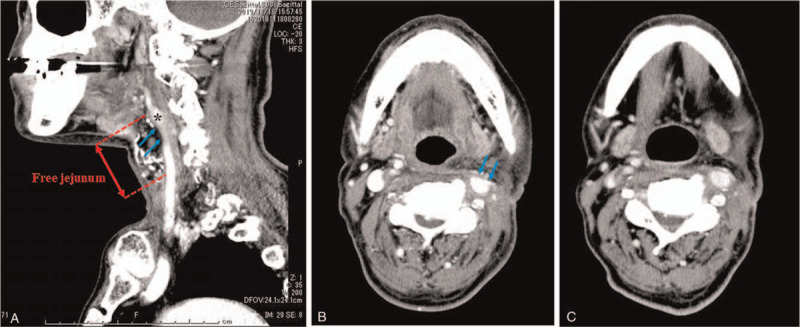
Head and neck CT at the carotid sinus level (27 d after the first surgery). After pharyngolaryngoesophagectomy with free jejunum replacement, the carotid artery is slightly compressed by the edematous free jejunum graft (Blue arrows) (A, sagittal view; B, axial CT). After the surgical release and re-anastomosis of the pharyngoesophageal stenosis via an open-neck approach, the free jejunum edema improved. The contact between the left carotid sinus and the free jejunum is reduced (78 d after the first surgery) (C). ∗Indicates the carotid sinus. CT = computed tomography.
